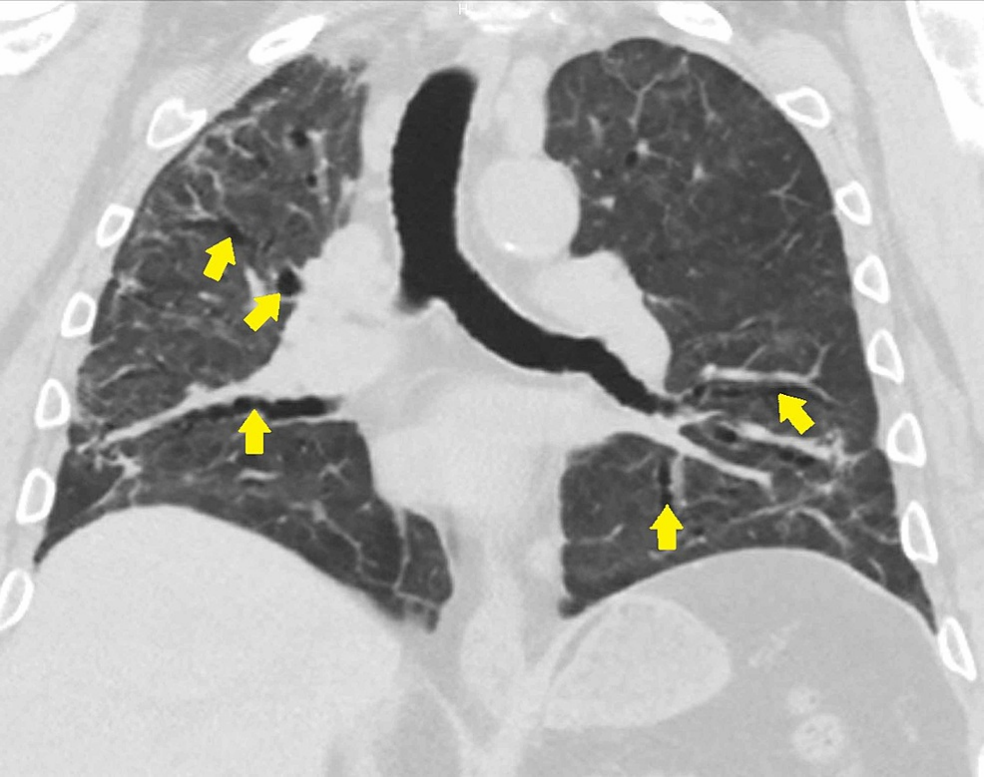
High-resolution CT of the chest (coronal view) at week 16: after five weeks of pirfenidone therapy, there is mild bronchiectasis. There is no reticular nodular density or significant ground-glass opacity. Yellow arrows show bronchiectasis
